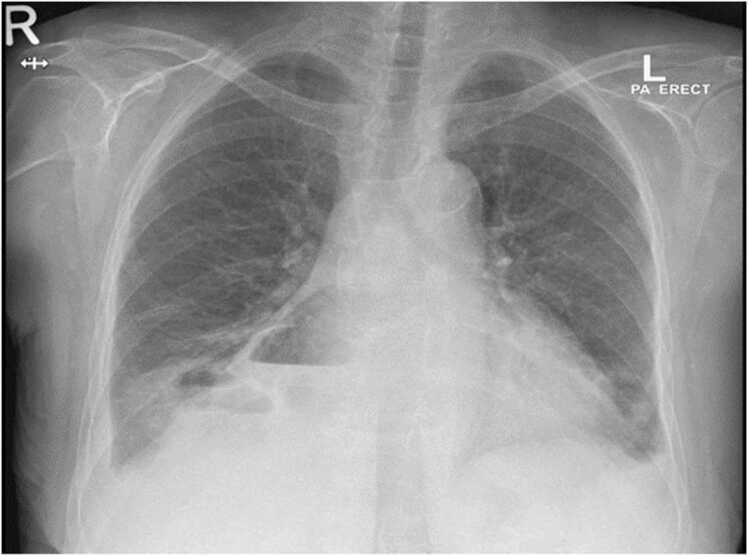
Posteroanterior chest Xray showing cavitary lesions with multiple air fluid levels silhouetting the right heart border and right hemidiaphragm accompanied by a mild right-sided pleural effusion.
